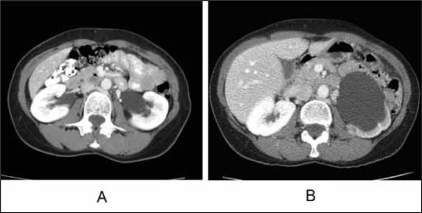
A. Initial CT scan. Note mild fullness of left renal pelvis. B. CT scan one year later. Note marked hydronephrosis of left kidney with thinning of renal parenchyma.
